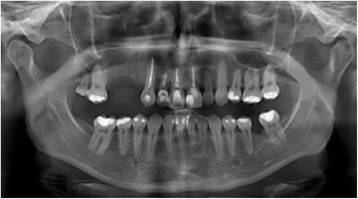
Panoramic radiograph 7 days postoperatively
